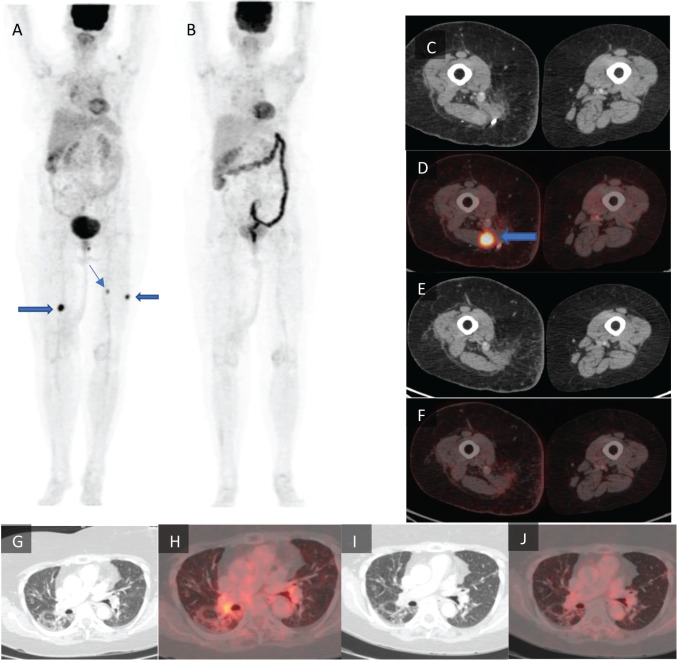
Maximum intensity projection images of (a): the pre-treatment PET-CT scan and (b): post-treatment PET-CT scan with the lower limb tumour lesions denoted by arrows. (c): Axial CT and (d): axial fused PET-CT of the pre-treatment scan with a lower limb tumour lesion denoted by arrow which is not seen in (e): the post-treatment axial CT and (f): axial fused PET-CT images of the same section. (g): Axial CT and (h): axial fused PET-CT images of the pre-treatment scan of the lungs in lung window. (i): Axial CT and (j): axial fused PET-CT of the same section of lungs in lung window.
